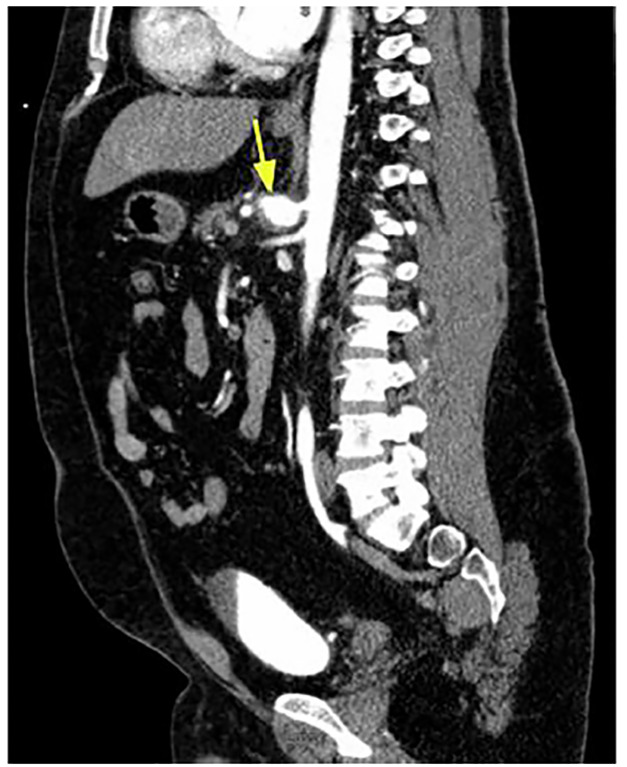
Sagittal view of computed tomography angiogram of abdomen and pelvis showing a proximal celiac artery aneurysm (arrow) with surrounding inflammation and trace hemorrhage.
